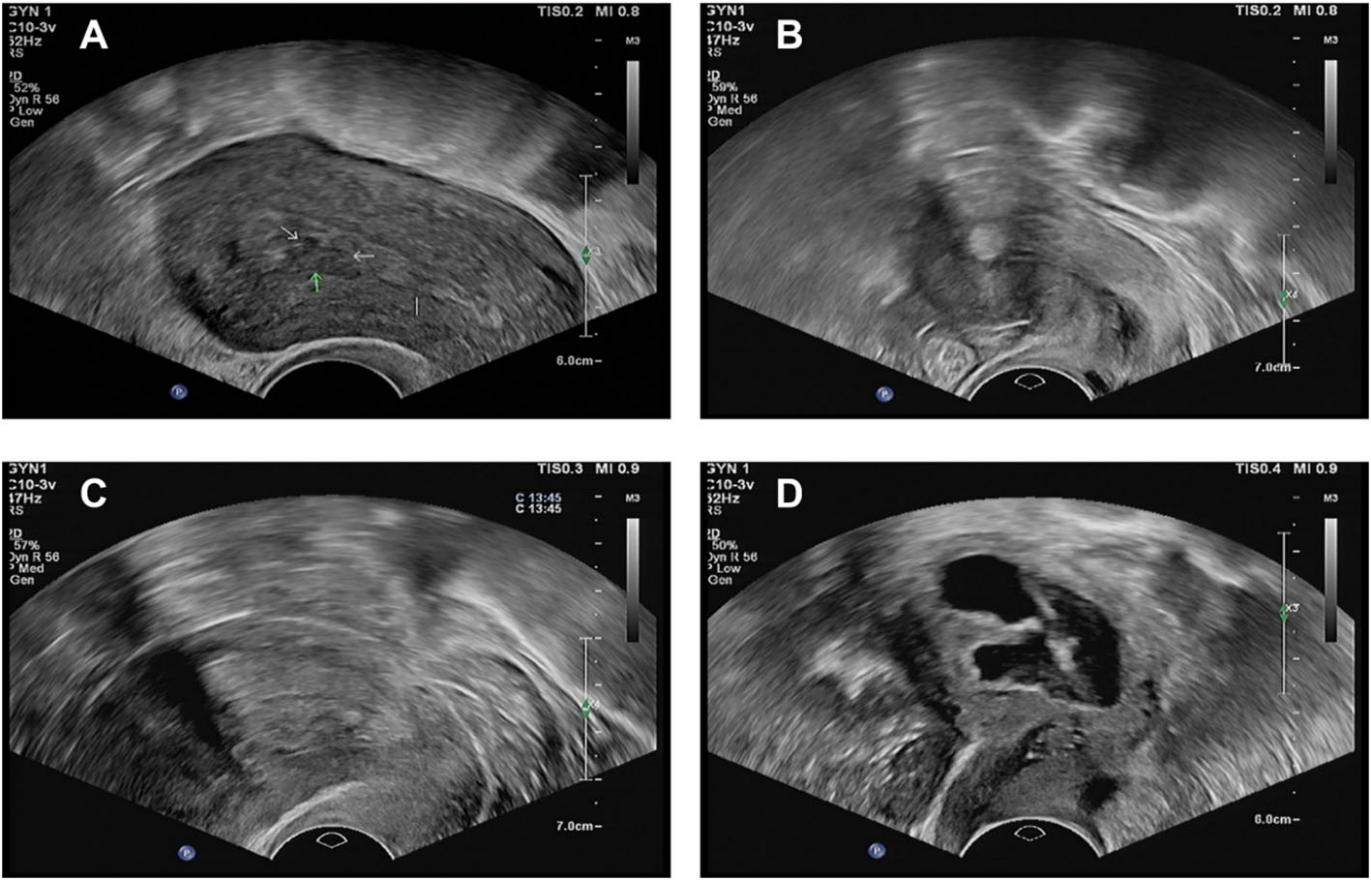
Ultrasonographic images of non-uniform endometrial echogenicity. (A) Focal non-uniform echogenicity. The image reveals a well-defined focal area within the endometrium exhibiting non-uniform echogenicity, contrasting with the relatively homogeneous surrounding endometrial background. This sonographic appearance is consistent with focal lesions, such as an endometrial polyp. (B) Focal non-uniform echogenicity (with cystic areas). The image demonstrates a focal, roundish lesion displaying characteristic non-uniform echogenicity, accompanied by anechoic cystic areas. The lesion is clearly demarcated from the myometrium. These findings suggest a focal endometrial lesion, such as an endometrial polyp. (C) Diffuse non-uniform echogenicity. The image shows diffuse thickening of the endometrium with entirely non-uniform echogenicity throughout the endometrial layer. The endometrial architecture appears disorganized with loss of homogeneity. No distinct focal mass is identified. This pattern of diffuse non-uniform echogenicity raises consideration for conditions such as diffuse endometrial hyperplasia. (D) Diffuse non-uniform echogenicity (with irregular background). The image depicts diffuse endometrial involvement characterized by non-uniform echogenicity, predominantly featuring a chaotic, heterogeneous background. The endometrial-myometrial junction is ill-defined. This sonographic presentation aligns with the features of diffuse endometrial pathology.
